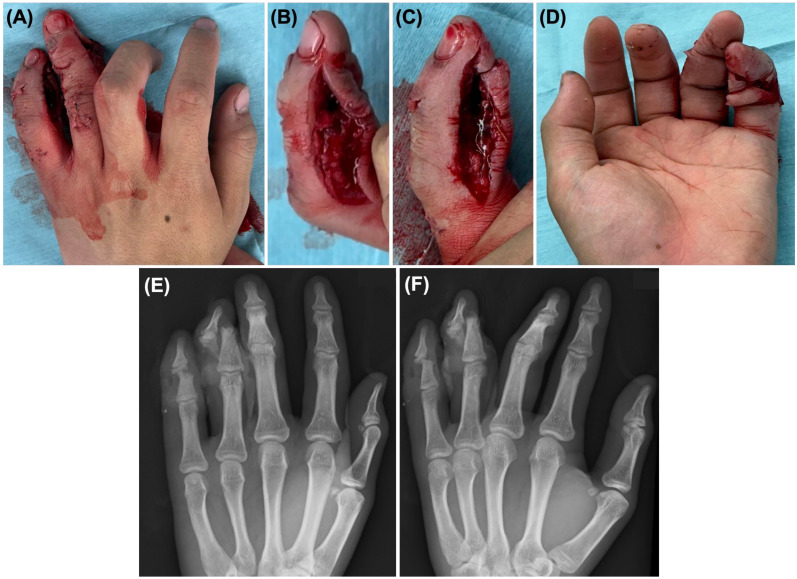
Preoperative photographs and radiographs of the left hand. (A) Dorsal view showing open injuries involving the ring and little fingers. (B) Radial-side view emphasizing ring finger injury. (C) Radial-side view emphasizing little finger injury. (D) Palmar view. (E) Posteroanterior radiograph and (F) oblique radiograph showing associated phalangeal fractures.
